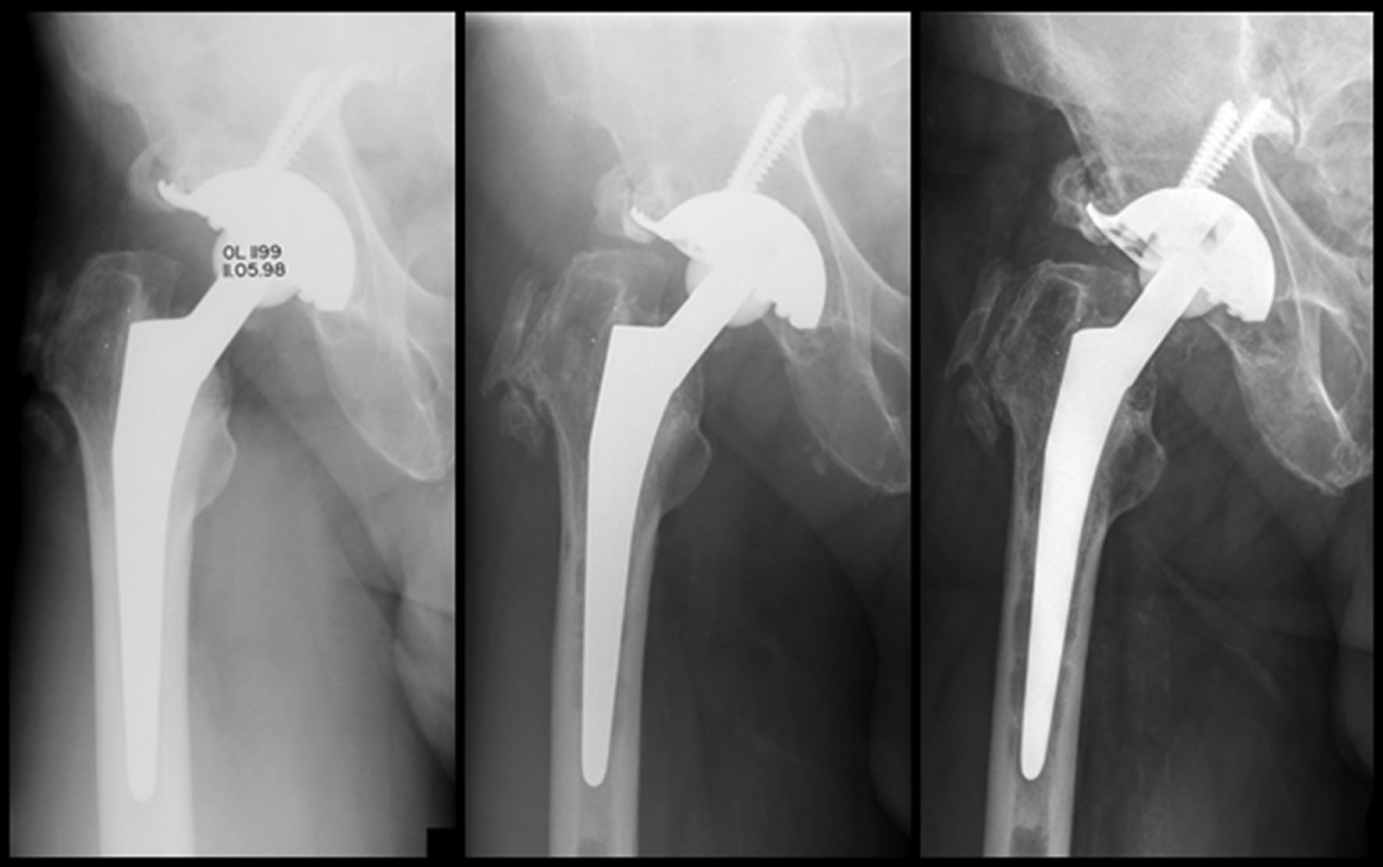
Cemented primary stem (Virtec stem and cementless SL-II cup) of a 86-year-old man, revision due to aseptic loosening. Radiographic follow-up 1 year (a), 10 years (b) and after 15 years (c) postoperative
